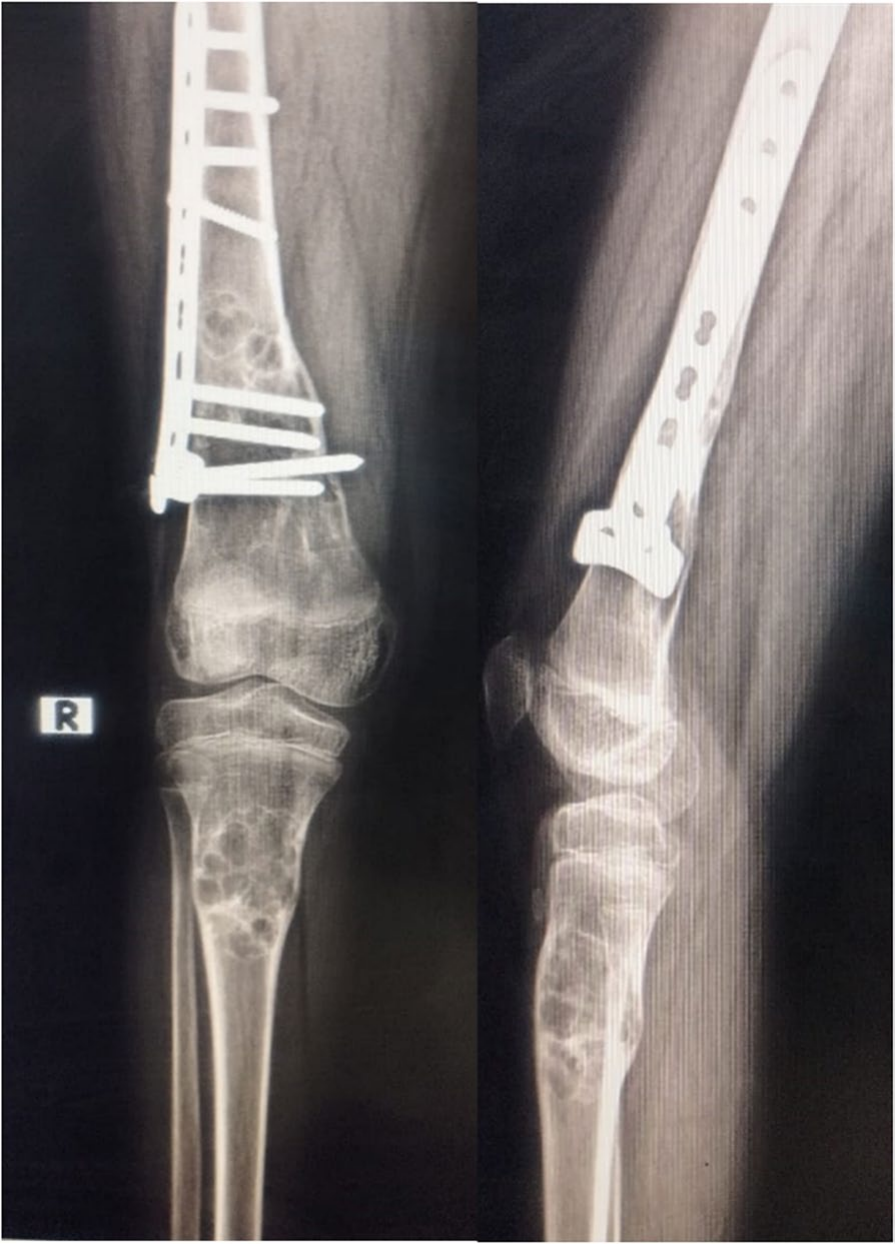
Postoperative xray showing union and calcification of the NOF’s
